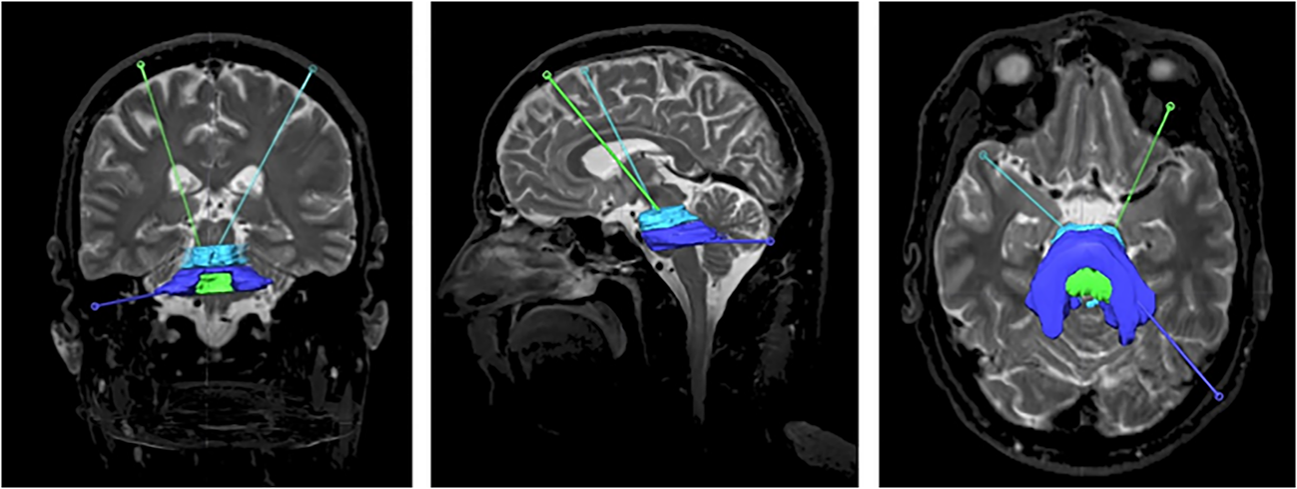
Posterior coronal (left), sagittal (middle), and axial (right) T2-weighted image showing the proposed pons regions of interest (ROI) and the preferred trajectory (light blue, upper pons; blue, pons at the level of the middle cerebellar peduncle; green, pons tegmentum)Medullary lesions can be approached through a supratentorial transfrontal approach. Alternatively, in cases of a lesion located at the ponto-medullary junction, a transcerebellar-transpeduncular approach can be evaluated (Fig. 6). Selected superficial medullary lesions could also be biopsied through an open transcisternal approach.
